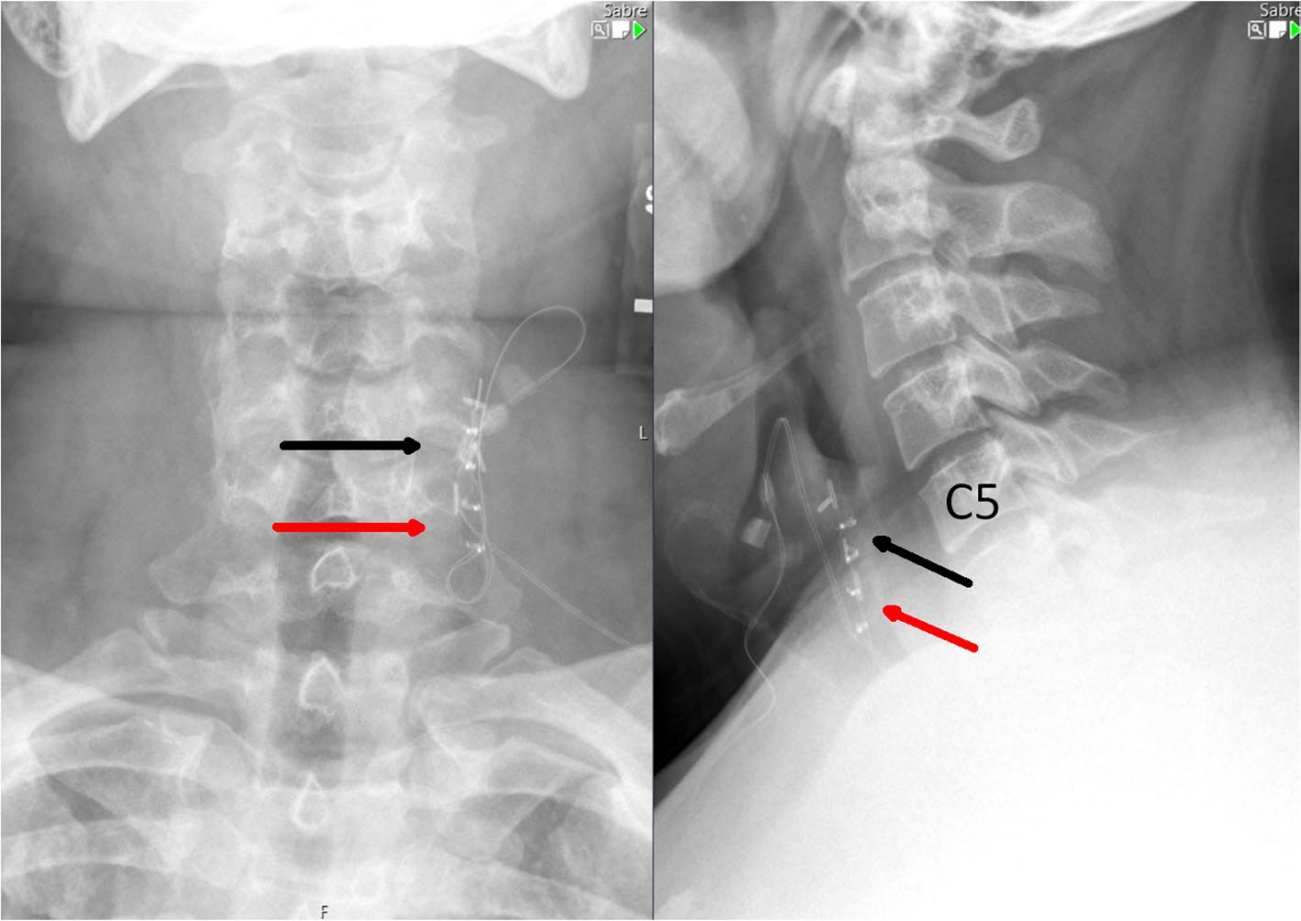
Postoperative plain radiograph of the neck demonstrating the retained old VNS electrode helical contacts (black arrows) at the C5 level as well as new VNS electrode helical contacts (red arrows) positioned more caudally
